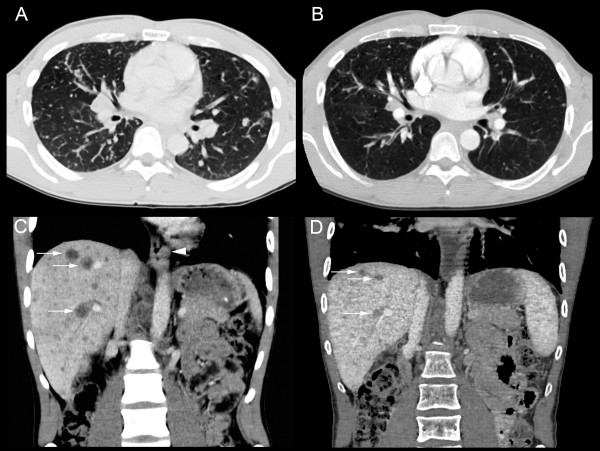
CT scans before and after the first three cycles of chemotherapy. (A) Chest CT scan at the time of diagnosis revealing multiple metastases in both lungs, and (B) the corresponding image after chemotherapy. (C) Coronal section from an abdominal CT scan at the time of diagnosis showing multiple liver metastases (three are marked with arrows) and also the tumor of the left wall of the gastroesophageal junction (arrowhead). (D) Corresponding section as C in an abdominal CT scan after chemotherapy showing decreased size of liver metastasis.
